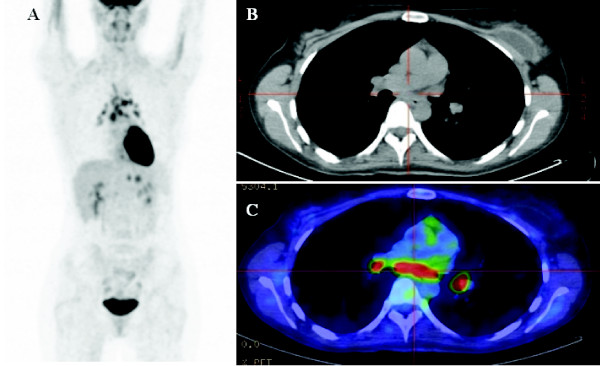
Posttreatment ^18^F-fluorodeoxyglucose (^18^F-FDG) positron emission tomography/computed tomography scan showing resolution of ^18^F-FDG-avid lymphadenopathy in the left cervical and supraclavicular nodes but new bilateral ^18^F-FDG-avid pulmonary hilar and mediastinal lymphadenopathy.
